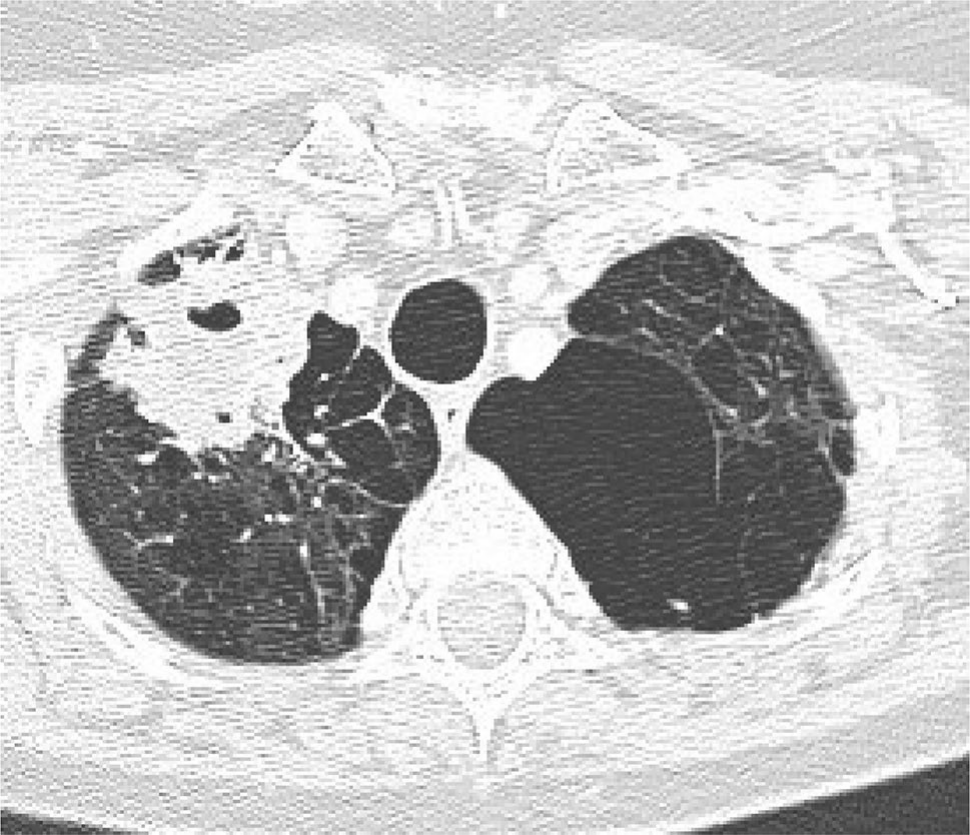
CT thorax of a female patient with asthma and significant COPD. A right upper lobe mass was found consistent with acute pneumonia. Evolution over the next few weeks and further imaging demonstrated the interval development of a fungal ball with an area of consolidation. This was consistent with a primary community-acquired *Aspergillus* pneumonia and the secondary development of chronic cavitary pulmonary aspergillosis with a fungal ball
